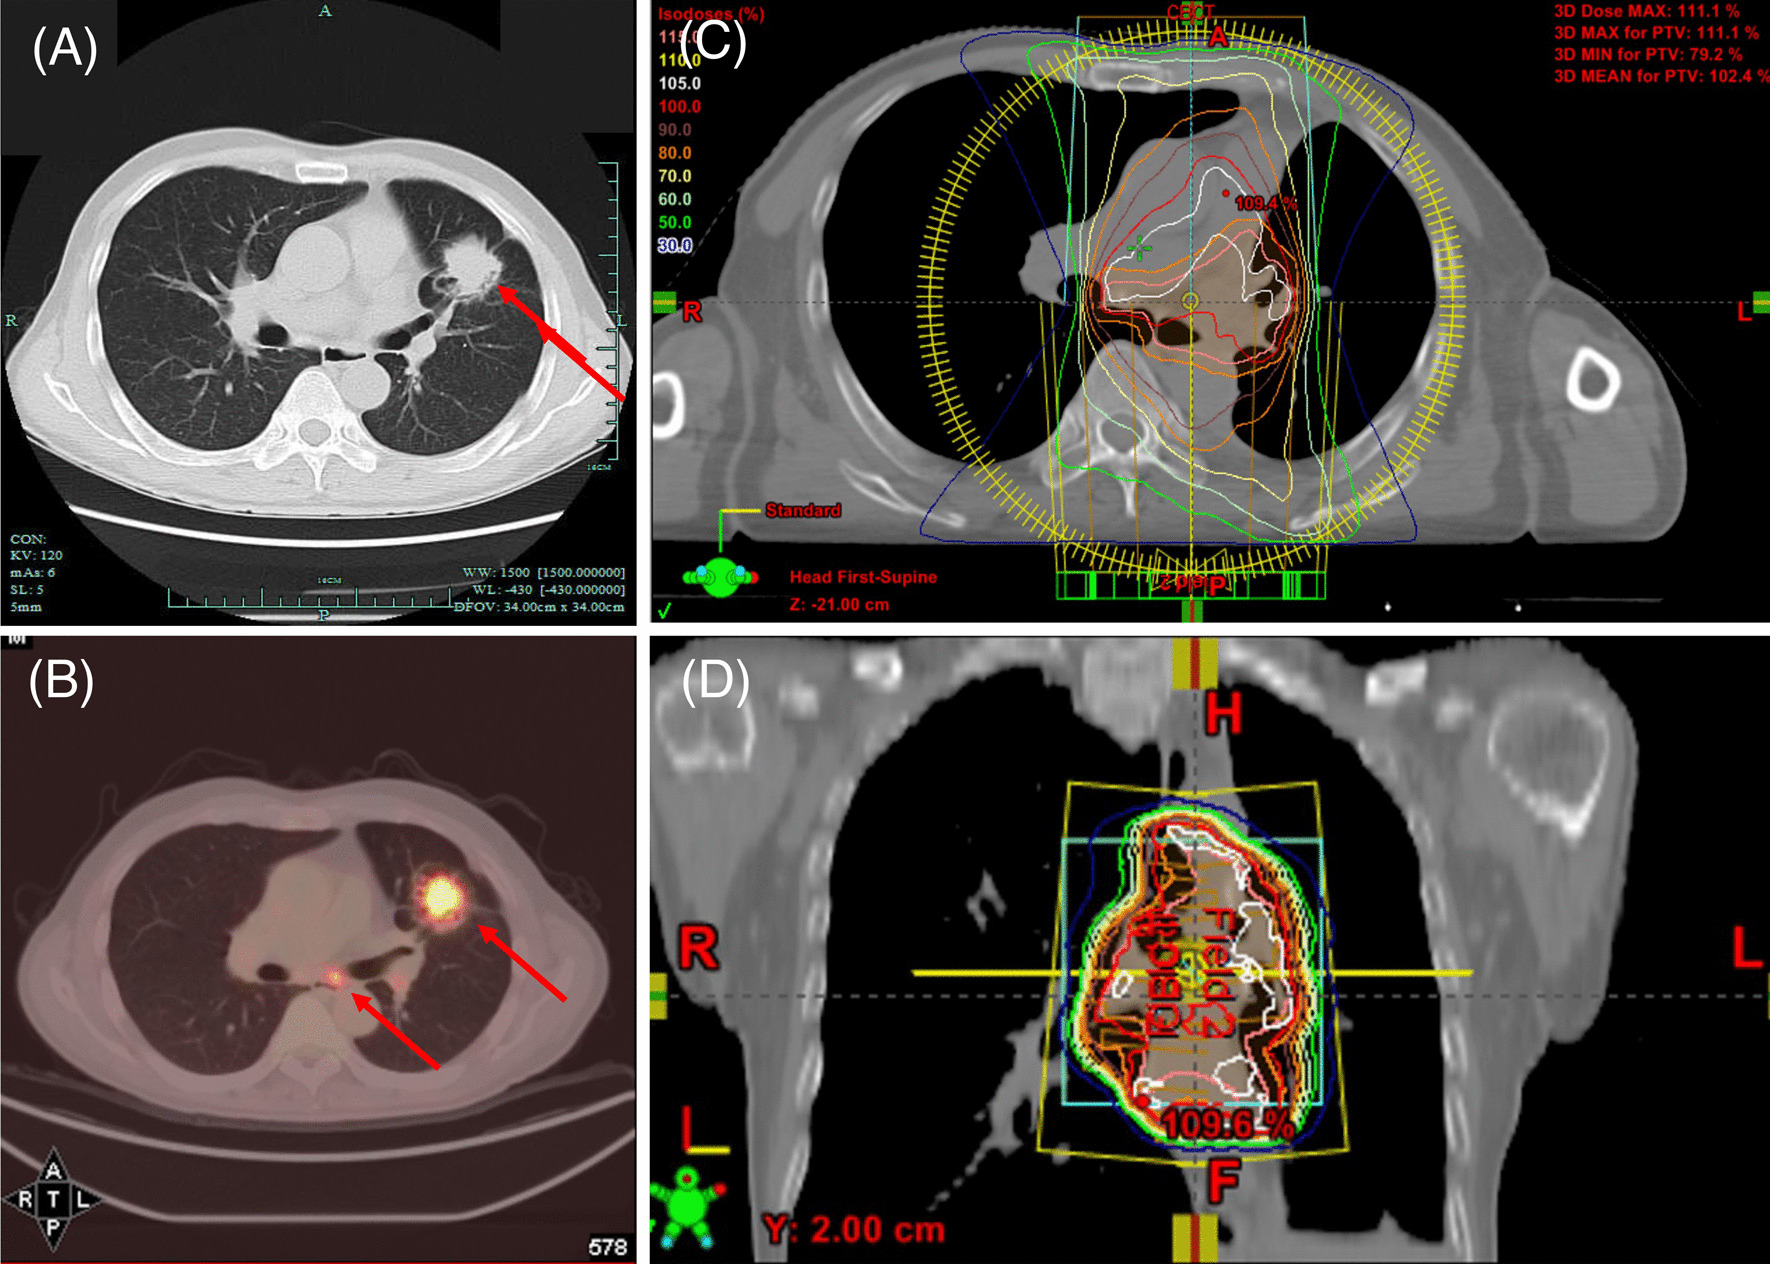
A CT scan of lung window captured on August 6th, 2016; B PET-CT captured on August 8th, 2016; C, D Radiation dosimetry post-operation. Significant abnormal findings noted (arrow)
